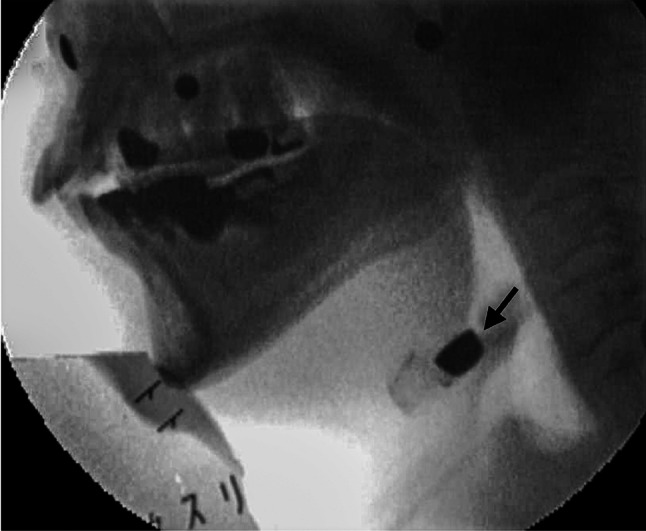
Radiograph, lateral view, showing pill residue in the epiglottis. The arrow indicates the pill residue.
